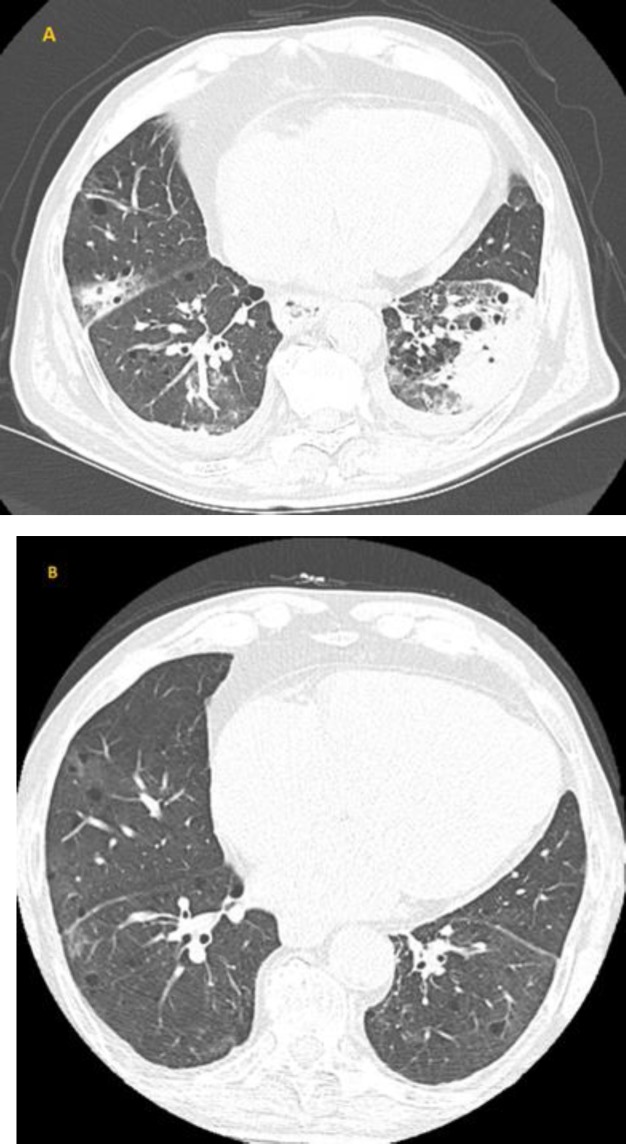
(A) The first chest CT confirmed the presence of a left lower lobe consolidation and revealed other smaller areas of consolidation in the right upper and middle lobes. (B) A follow-up CT scan in 1 month demonstrates almost a complete clearance of the previous findings
